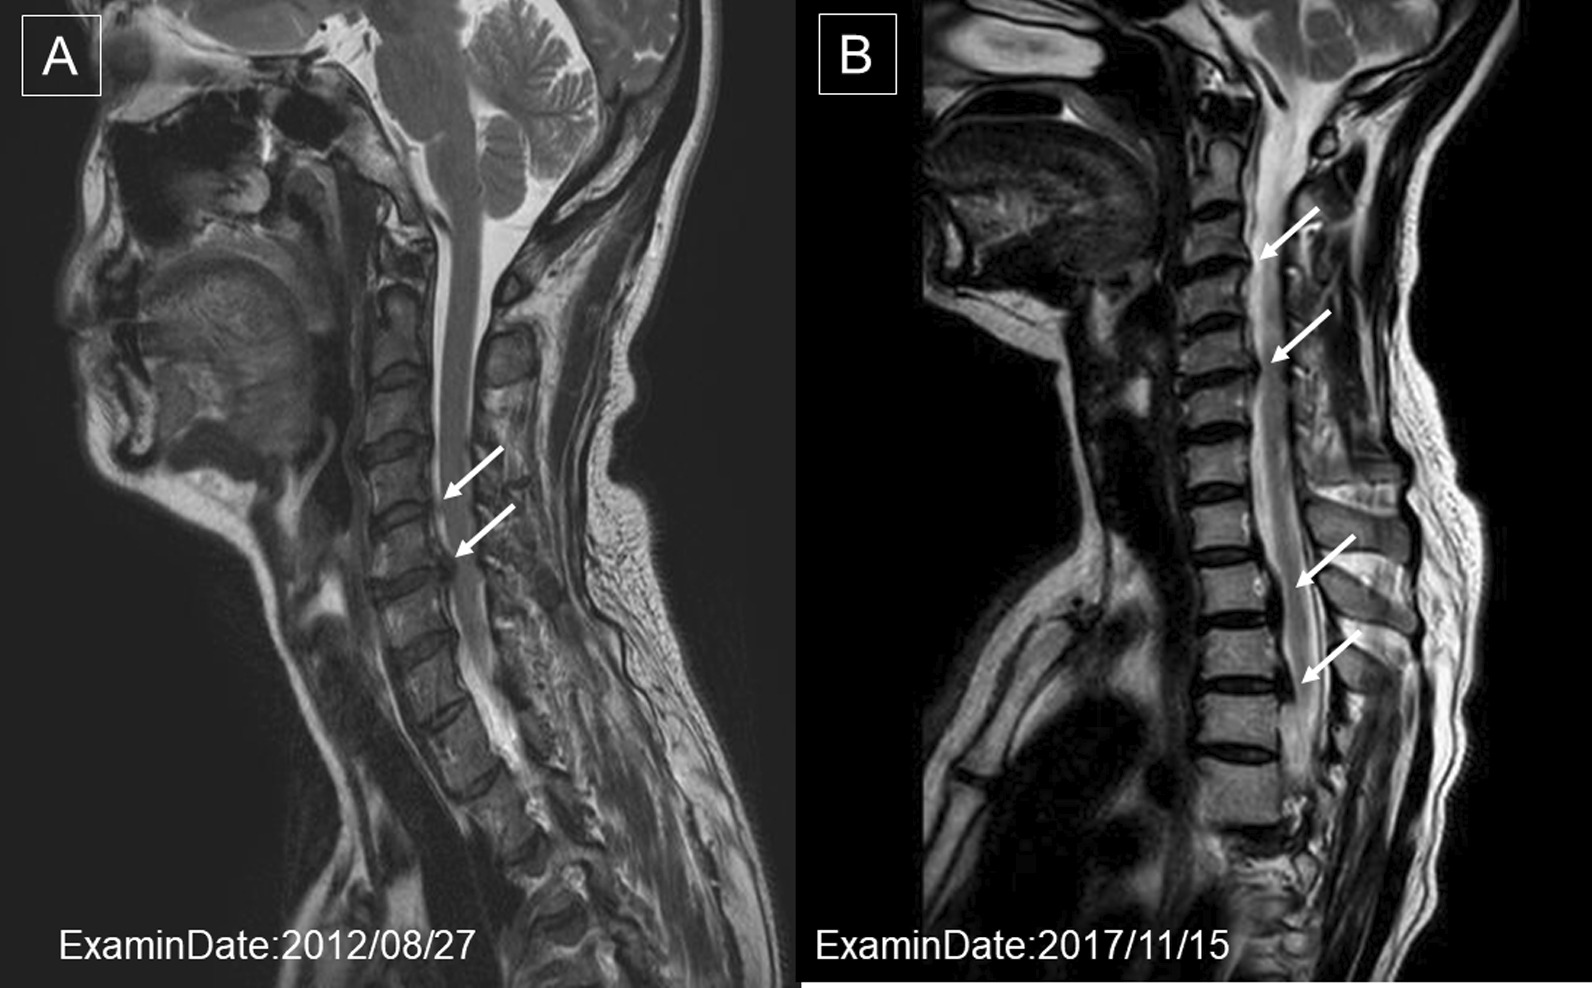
a Magnetic resonance image on which the definitive diagnosis of ossification of the posterior longitudinal ligament of the cervical spine on 27 August 2012, was based. The arrows indicate the ossification of the posterior longitudinal ligament of the third cervical spine. b Magnetic resonance image of the ossification of the posterior longitudinal ligament of the cervical thoracic spine on 15 November 2017. A new ossification of the posterior longitudinal ligament was confirmed in the fourth thoracic vertebra (arrows)
